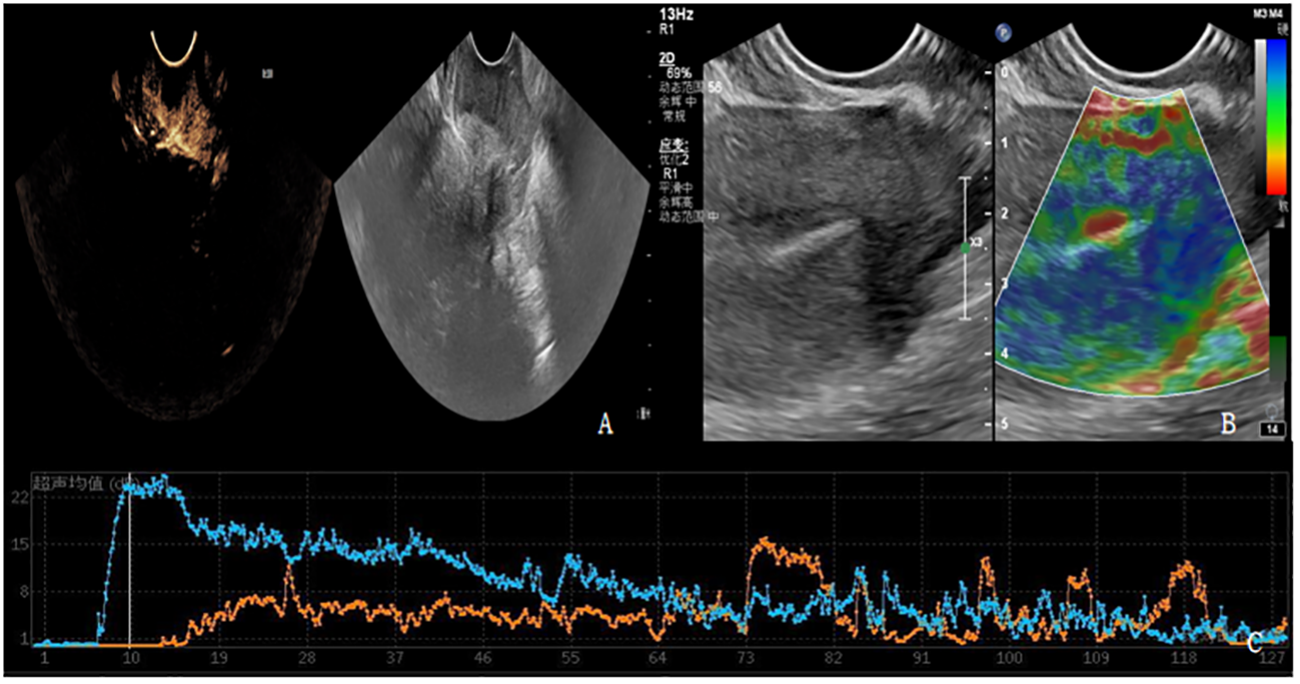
(A-C) shows contrast-enhanced ultrasound and ultrasound elastography of PD patients. (A) Lesions showed moderate to high degree of enhancement. (B) The lesion had stiff elasticity, the center was blue and green, and blue was the majority. The elasticity score was 4 points, and the SR value was about 3.8. (C) TIC curve drawn after focal angiography. Blue represents the TIC curve of the lesion, and orange represents the TIC curve of normal tissue at the same level of the lesion. The lesion PI was 22dB, TTP was 10S, and AUC was 1124dB.S.
